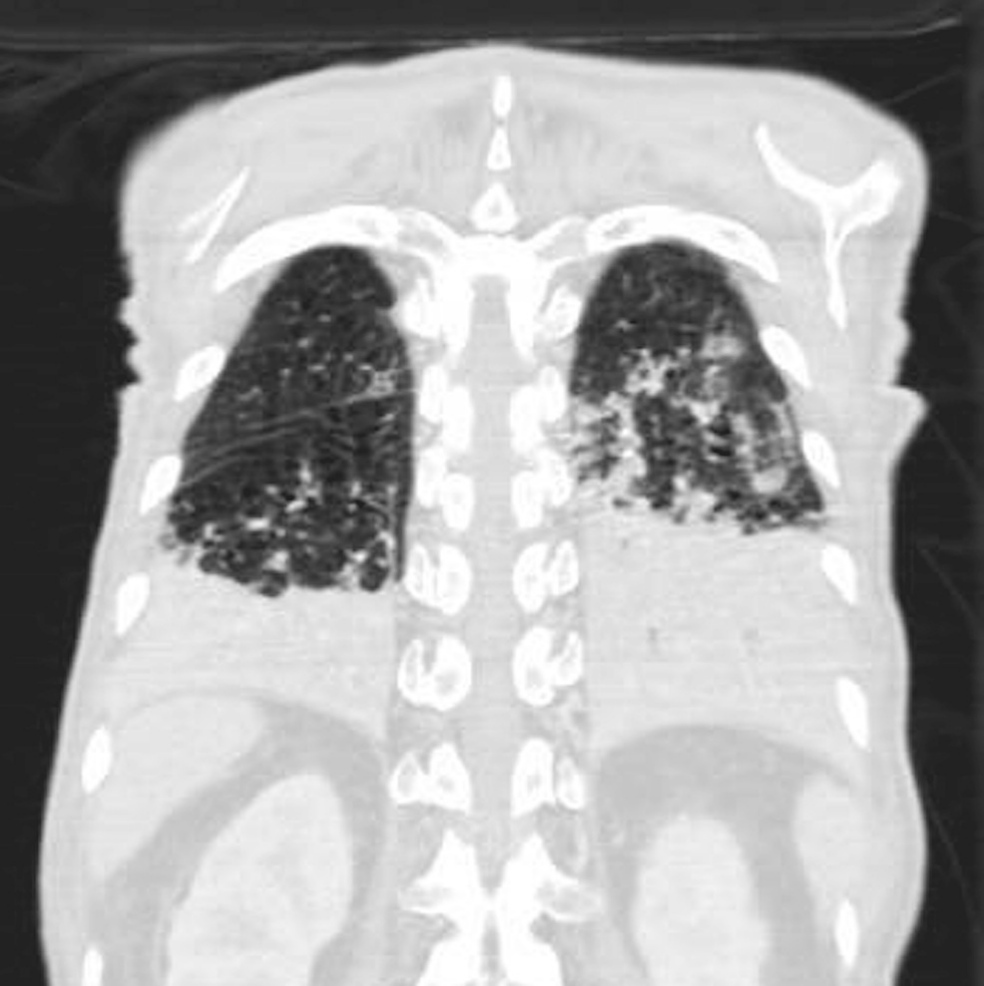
Coronal contrast-enhanced chest CT scan showing bilateral pulmonary inferior lobe consolidation.
